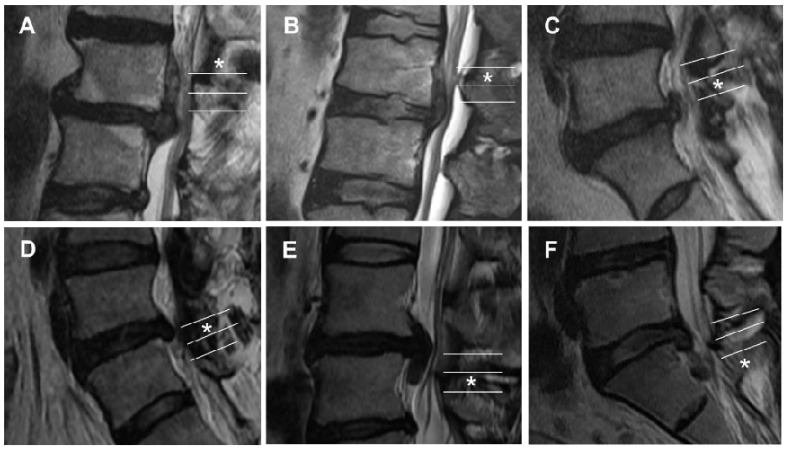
Grade of migrated lumbar disc herniation on a sagittal MRI image (asterisk). (A) Grade 1, superior very high grade. (B) Grade 2, superior high grade. (C) Grade 3, superior low grade. (D) Grade 4, inferior low grade. (E) Grade 5, inferior high grade. (F) Grade 6, inferior very high grade.
